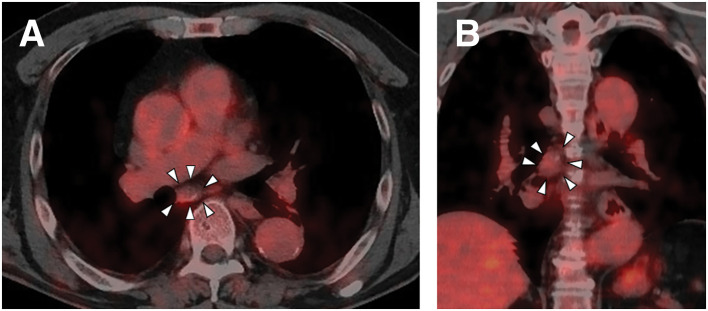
PET showing the mild FDG uptake (SUVmax 2.5) of the mass (arrowheads). (A) Axial PET image. (B) Coronal PET image. FDG, fluorodeoxyglucose; SUVmax, maximum standardized uptake value
